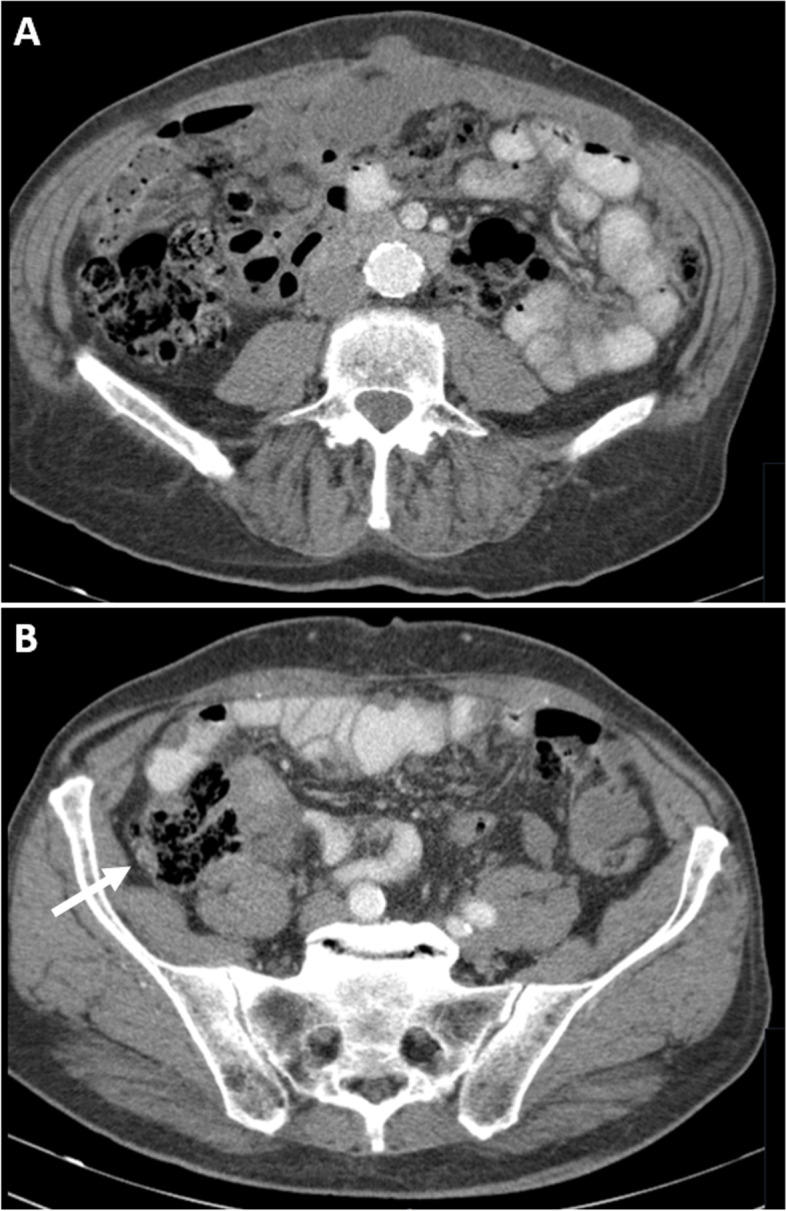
Abdominal CT scans of initial presentation. Cross-sectional images showing mucinous ascites and tumour infiltration of the mesentery and abdominal wall (A), and an indistinct cecum mass, indicated by the white arrow (B)
